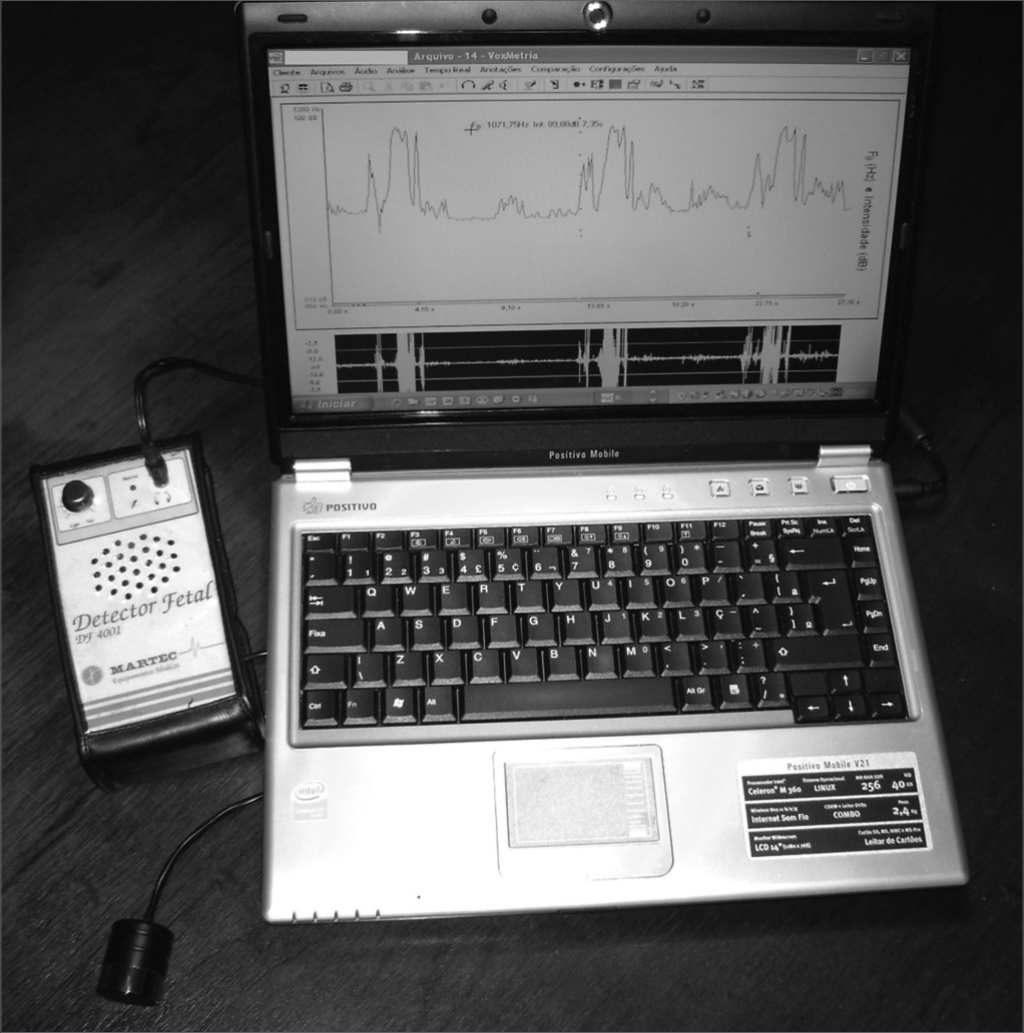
Doppler sonar coupled to a notebook computer
